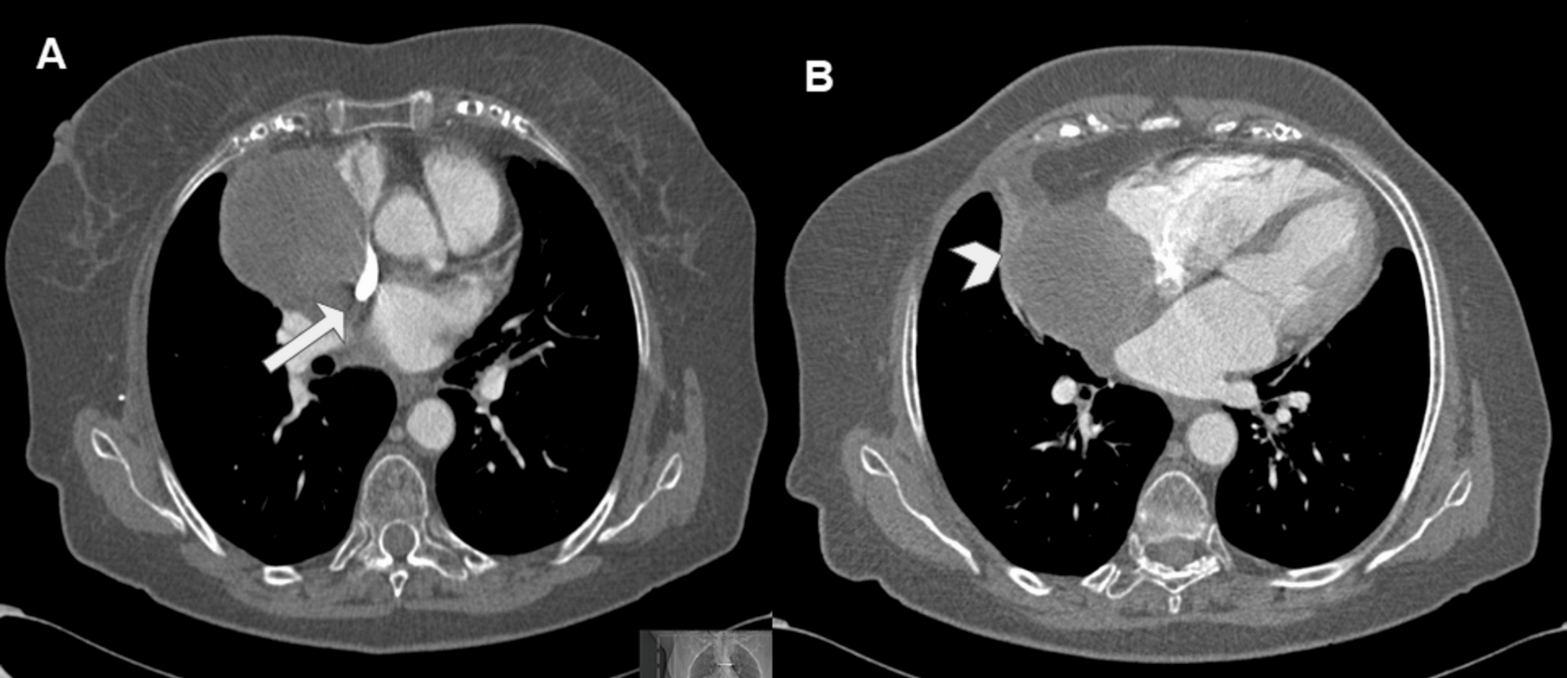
Contrast-enhanced CT in axial view at the four chambers (A) and at the SVC levels (B). (A) CT shows a voluminous rounded mass occupying the right atrial lumen. It seems to originate from the posterior and lateral auricular walls. (B) It also indented and reduced the caliber of SVC (arrow). The mass showed heterogeneous density, with soft-tissue areas with contrast uptake in its medial region and areas with fluid density in its lateral portion (arrowhead). CT: computed tomography; SVC: superior vena cava.
